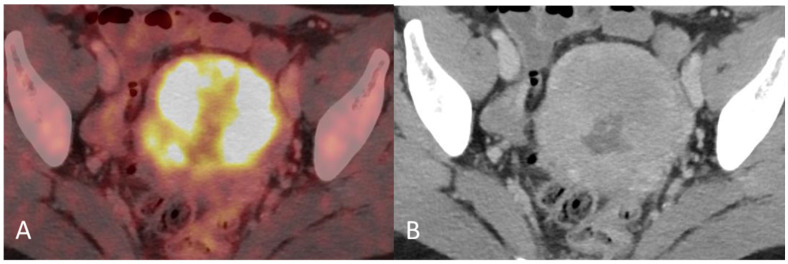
(A) Baseline axial PET/CT fused image. The metabolic activity is inhomogeneous, with areas of high to low metabolic activity. (B) Contrast-enhanced axial CT shows an enlarged uterus with endometrial thickening and polypoid tumour masses protruding into the uterine cavity. The tumor appears hypodense to the myometrium with signs of myometrial invasion. There was no involvement of the ovaries, fallopian tubes or cervix on either the PET or CT.
